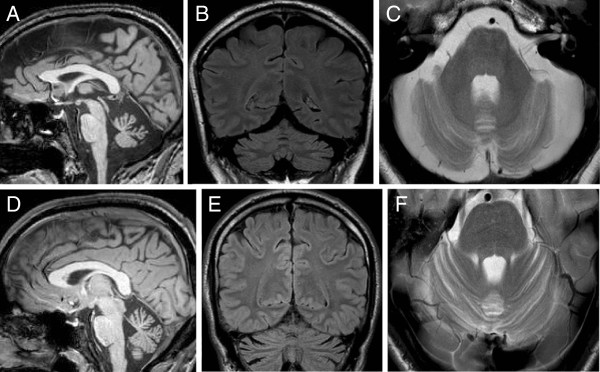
Brain MRI in AOA2 patients. Panels A-C show brain MRI images of patient the P641 with 36-year disease duration: (A) Mid-sagittal T1-weighted image showing marked atrophy of the vermis, and moderate atrophy of the midbrain; (B) Fluid attenuated Inversion Recovery Image (FLAIR), and (C) T2-weighted image confirm marked atrophy of the vermis and show atrophy of the cerebellar hemispheres without abnormal signal intensities of the cerebellar cortex. Panels D-F show an analogous sequence of brain MRI images from a younger AOA2 patient with 7-year disease duration (P2062). Note the moderate atrophy of the vermis and mild atrophy of the anterior portion of the cerebellar hemispheres without abnormal signal intensity. The midbrain was normal.
